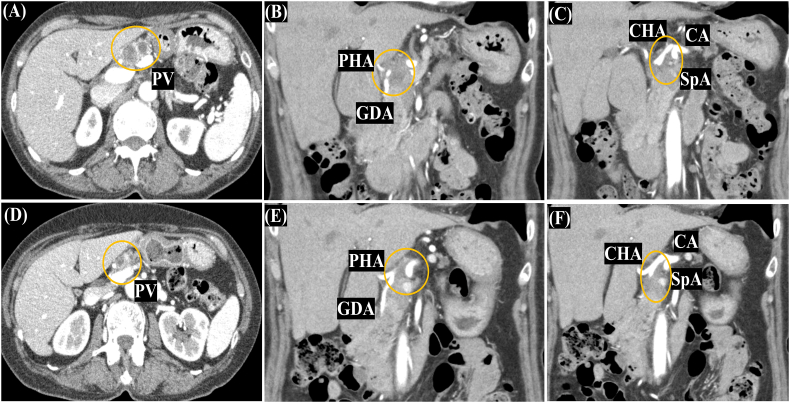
Abdominal CT: Pre-chemotherapy (A, B, C), post-chemotherapy (D, E, F). Computed tomography (CT) demonstrated a low attenuation solid mass on the PV/SMV (A, D) that is invading the GDA (B, E) and has an abutment with CA (C, F).
